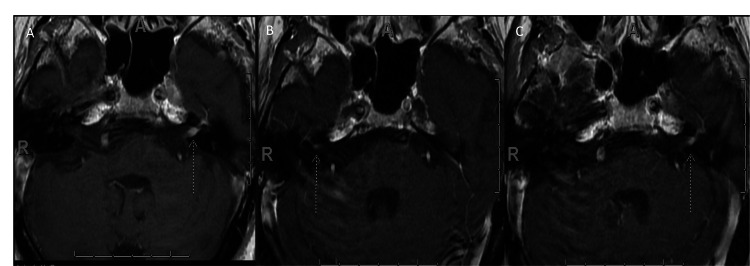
Initial brain MRI and repeat brain MRI. Initial brain MRI: T1 sagittal with gadolinium contrast shows left facial nerve enhancement (A). Repeat brain MRI: T1 sagittal with gadolinium contrast shows right facial nerve enhancement (B) and enhancement of the left internal auditory canal (C).
